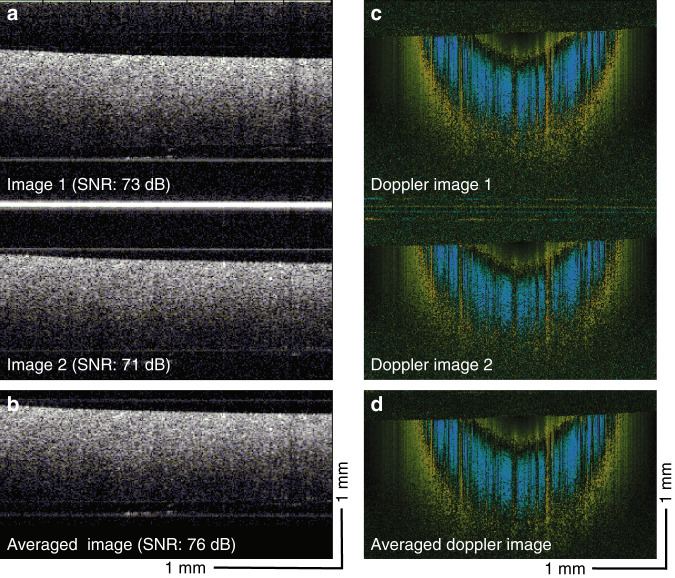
SNR quantification. a, b Original and averaged OCT images, respectively. c, d Original and averaged Doppler OCT images, respectively
